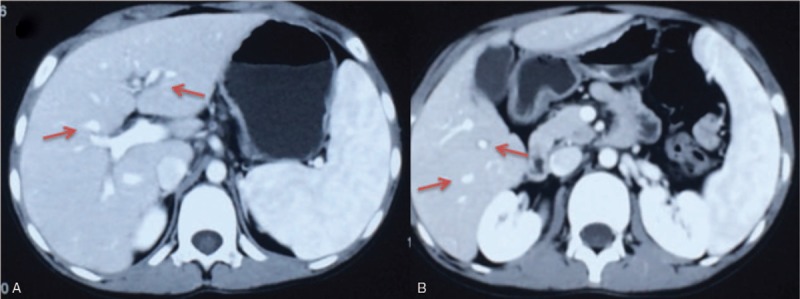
Hepatic computed tomography scan. Computed tomography scan shows a heterogeneous and nodular contour of the liver with mild splenomegaly.
